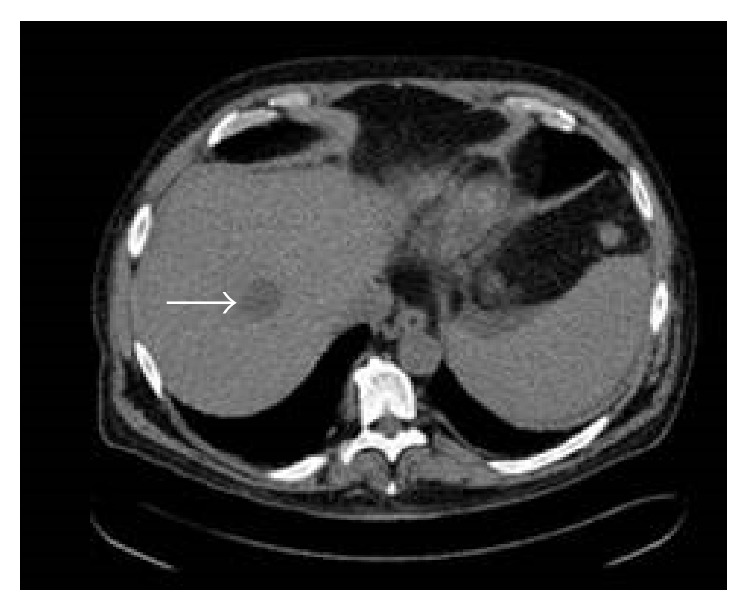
CT image on day 14 redemonstrated enlarged ill-defined hypodensity within the right hepatic lobe.
